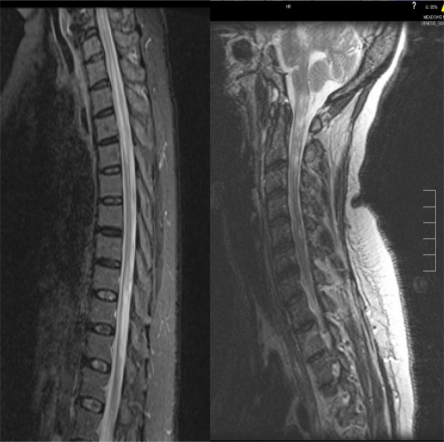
Fig. (2a) shows a T2 sagittal spine MRI of a 38 year-old woman who presented to our hospital with acute incomplete paraparesis displaying the typical LETM in acute NMO. Fig. (2b) shows a T2 sagittal spine MRI of a 51 year-old woman with chronic relapsing NMO showing the typical manifestations of chronic NMO including cord atrophy and patchy lesions.
